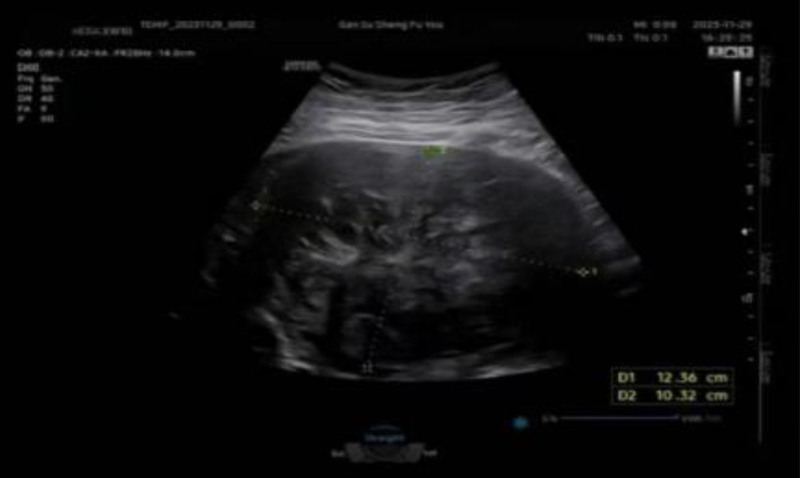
Obstetric ultrasound image at 36 weeks of pregnancy.
